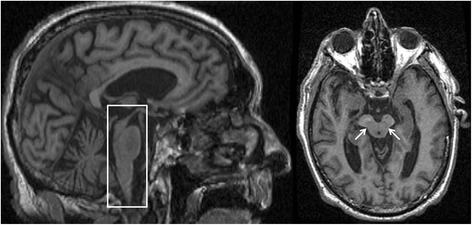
MRI of a patient with a clinical diagnosis of Progressive Supranuclear Palsy. The image on the left is a sagittal T1 sequence showing the ‘Hummingbird’ sign (box), while the axial T1 sequence on the right shows the ‘Morning Glory’ sign (arrows) both features seen in Progressive Supranuclear Palsy
